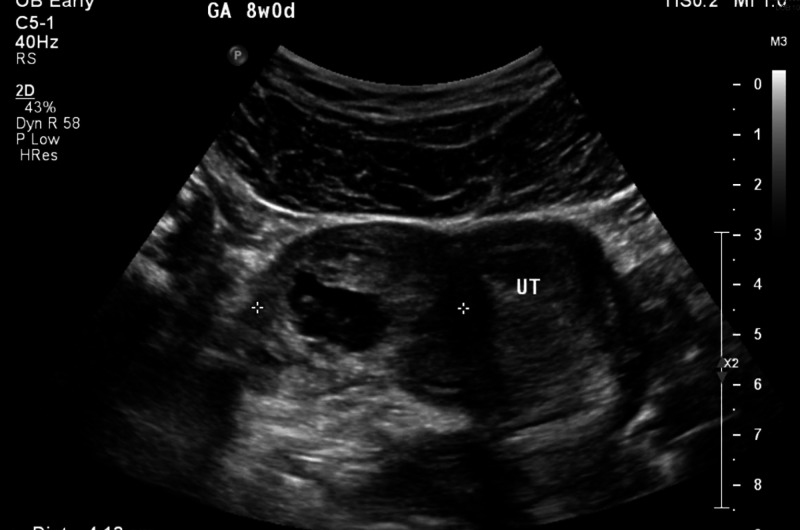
Day 1 ultrasonography (US) of cornual ectopic pregnancy in relation to the uterus (UT)
